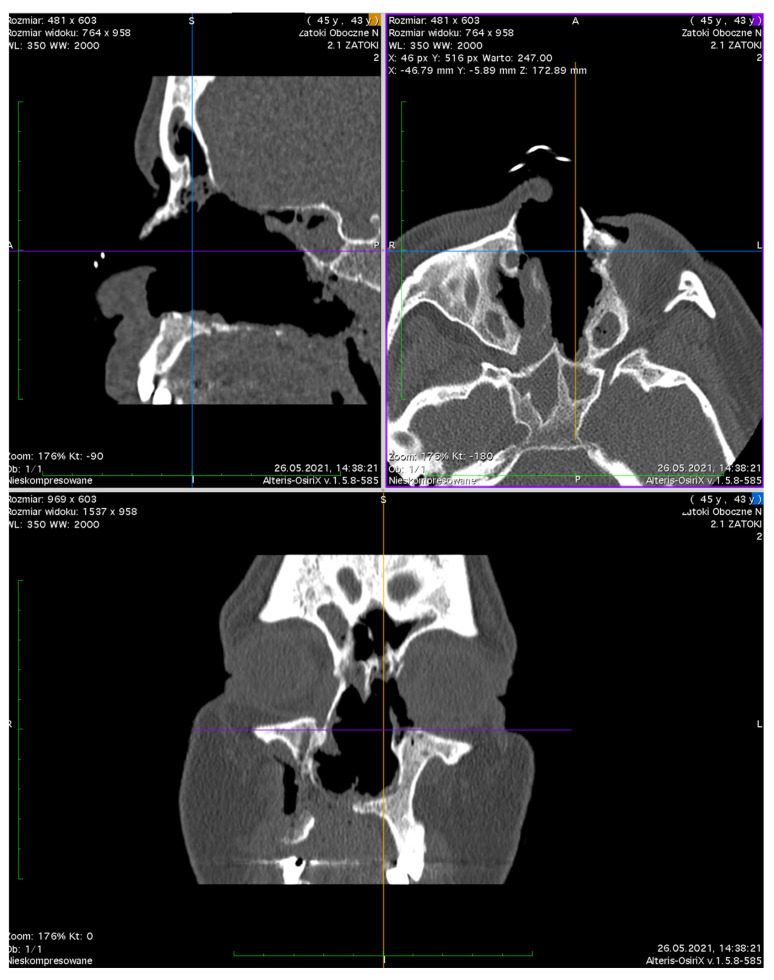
A CT scan of the head. Loss of bony and soft tissue in the middle face and left orbital walls.
